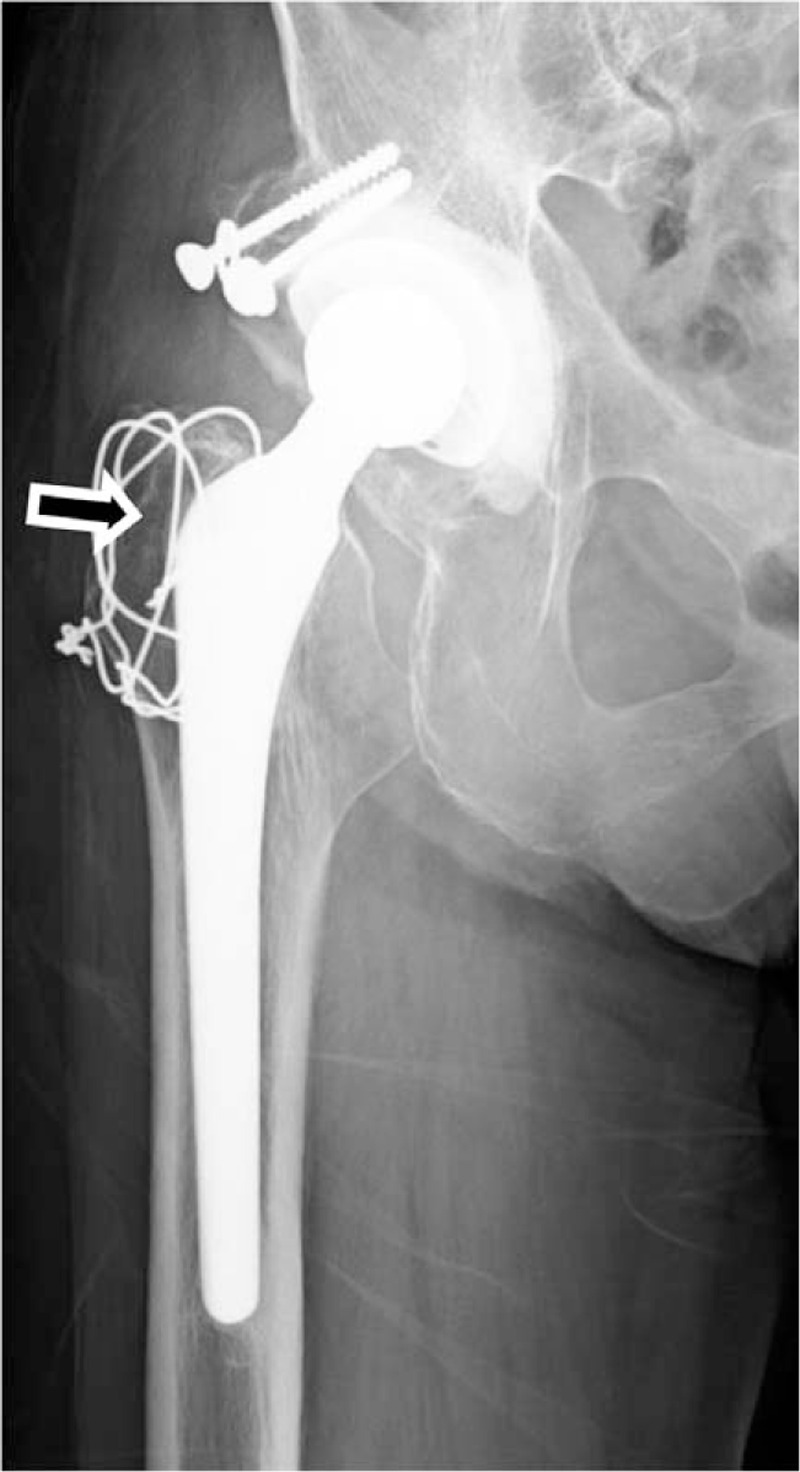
Radiological images. Plain radiograph of the right hip at the time of admission showing slight osteolytic changes at around the implant (black arrow), but no evidence of loosening of the implant.
